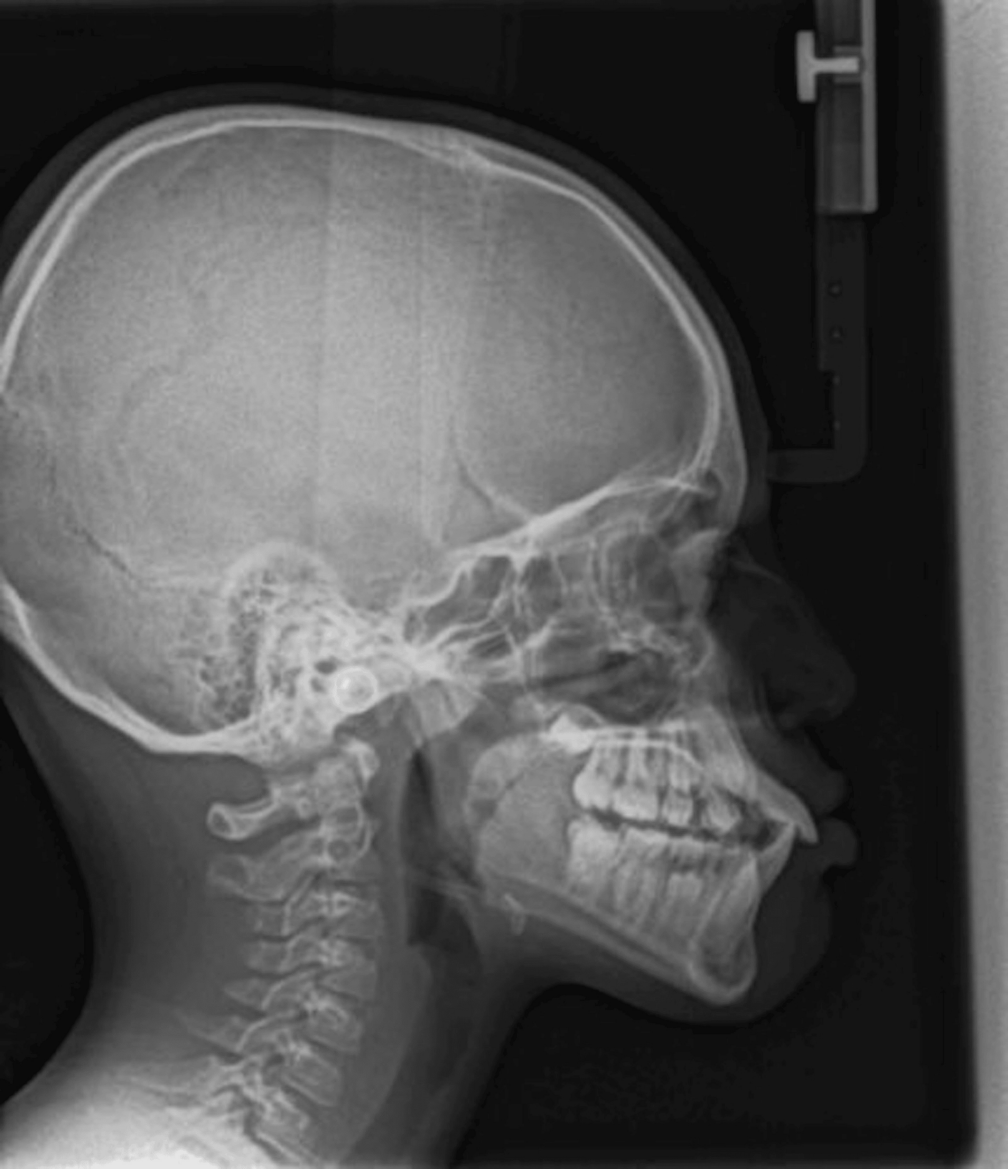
Cephalometry radiograph of male child patient in Figure 5 with bimaxillary proclination.
